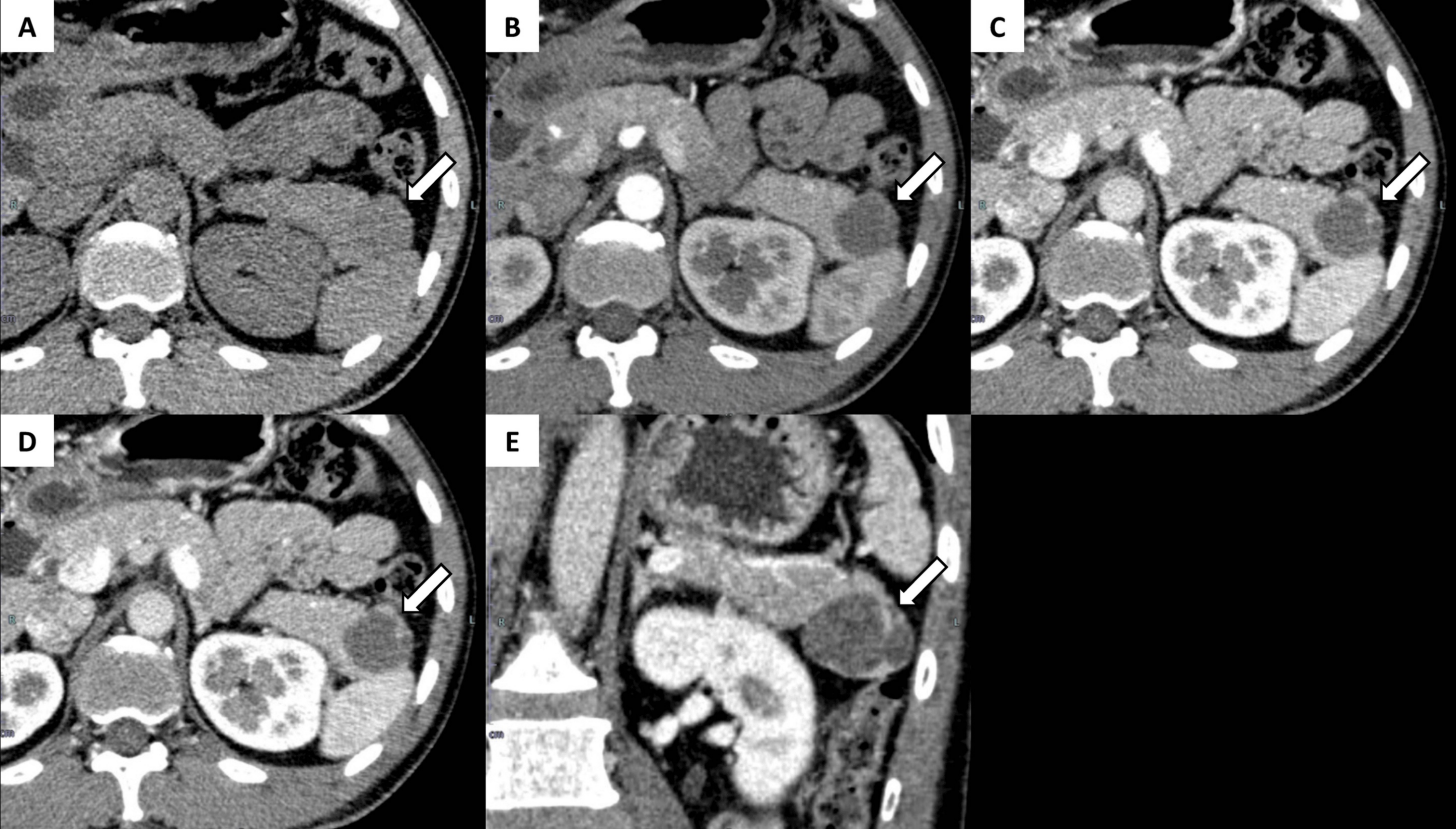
Computed tomography imaging findings (A) Unenhanced computed tomography scan reveals a hypodensity lesion in the pancreatic tail (arrow). (B-D) In the contrast-enhanced CT scan, the early arterial phase (B), late arterial phase (C), and portal phase (D) show a solid component surrounding the cyst, with a density similar to that of the spleen (arrows). (E) A coronal image of the late arterial phase shows that the pancreatic cyst measured 38 mm in diameter (arrow).
